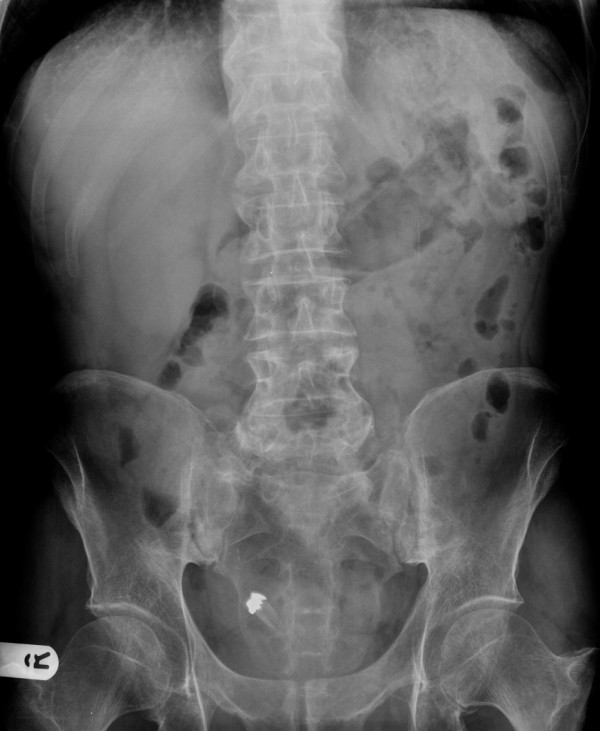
Abdominal x-ray showing the foreign body in right iliac fossa.
